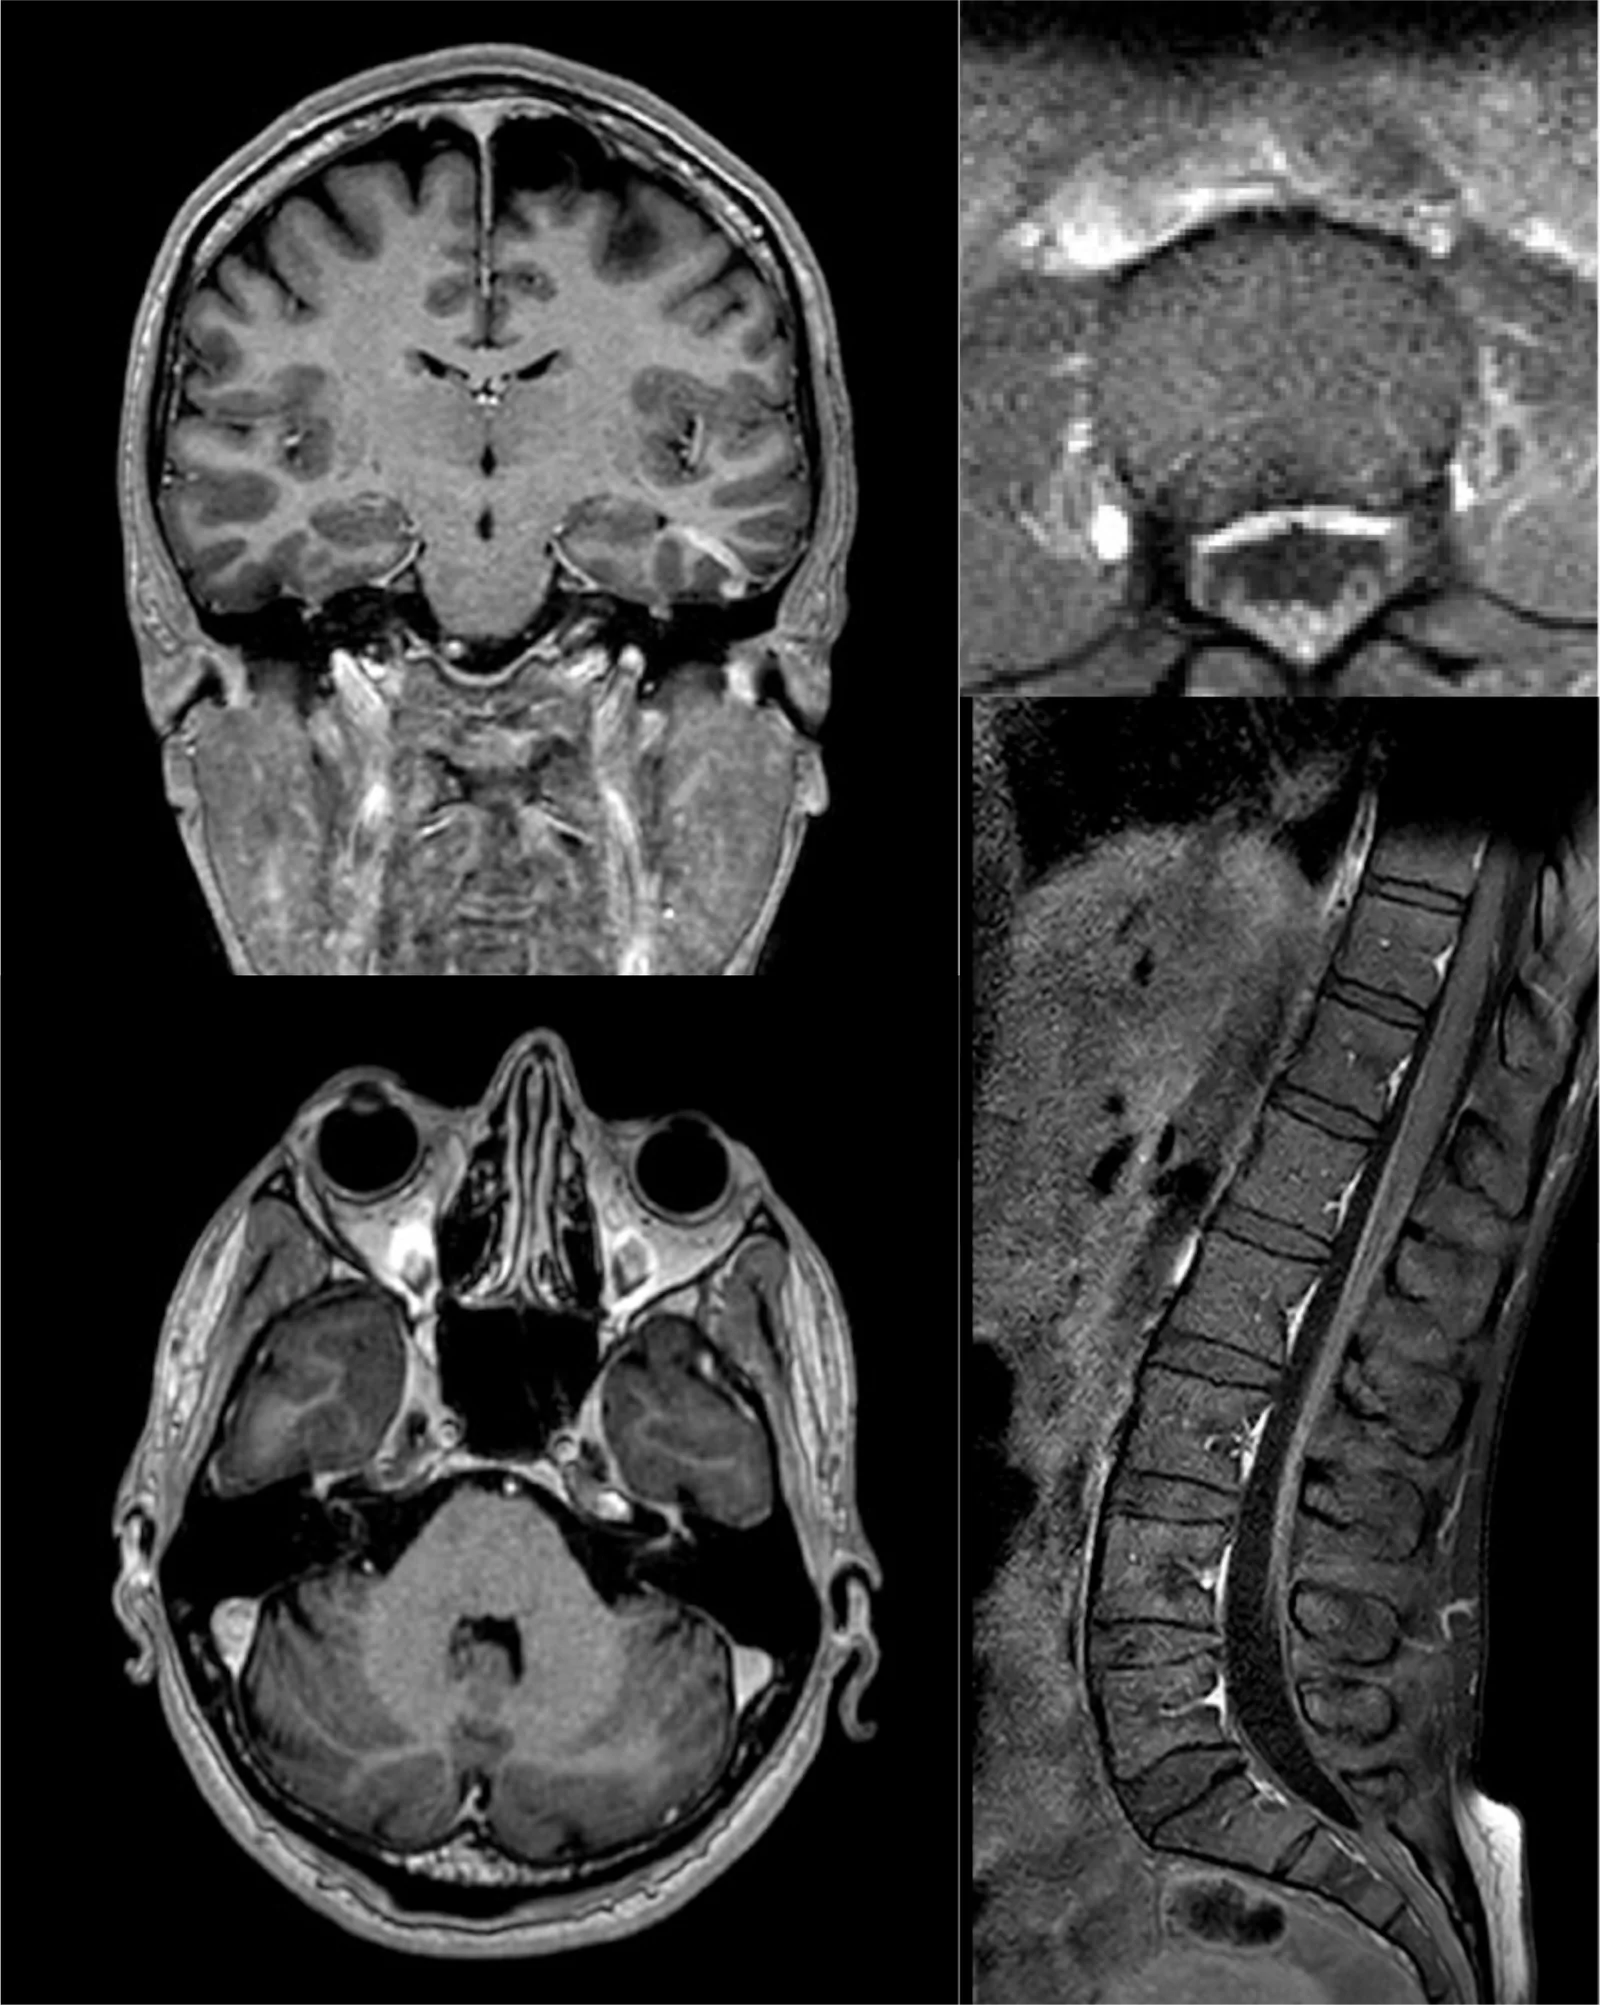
Contrast-enhanced MRI findings. Gadolinium-enhanced T1-weighted brain images in the coronal and axial planes show bilateral enhancement of the intracranial facial nerves; gadolinium-enhanced T1-weighted lumbar spine images in the axial and sagittal planes show enhancement of the cauda equina nerve roots.
